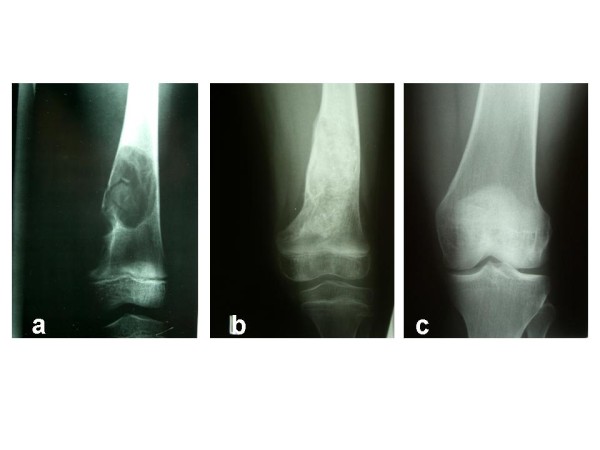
(a) 6-year-old female patient initially treated by injection of methylprednisolone. The picture shows the situation before the first injection. (b) 7 months later (3 month after revision curettage and bone grafting for pathological fracture of the distal femur in the course of corticoid therapy). (c) 16 years after the initial radiograph. No aspect of UBC is remaining.
